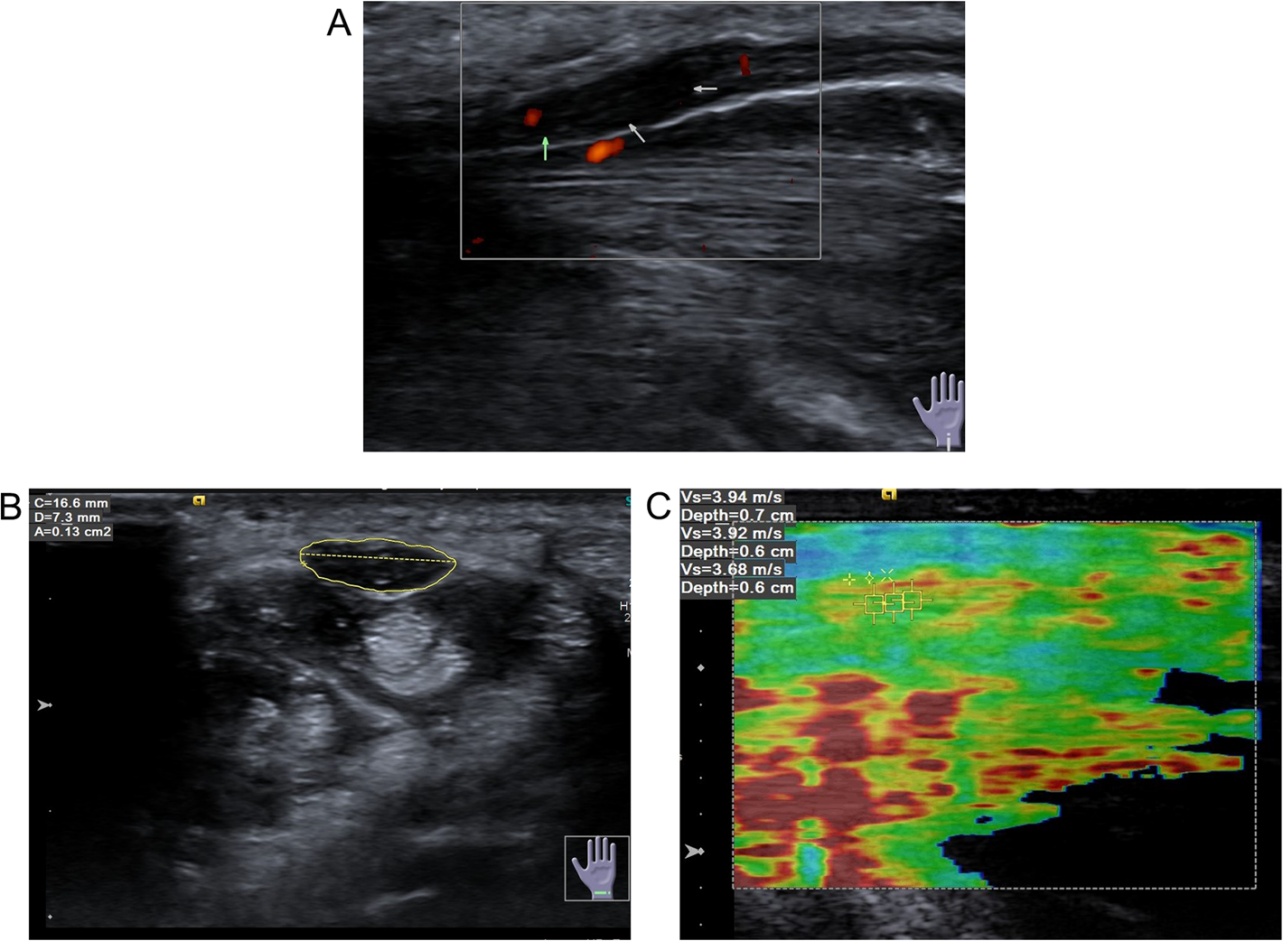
Ultrasound diagnosis (a) The longitudinal section of HFUS showed thickening of the middle normal nerve in carpal canal, decreased echo, and increased blood flow signal in power Doppler ultrasound; b Median nerve CSA was measured using the pisiform bone as a reference; c ROI was measured three times at the carpal tunnel inlet and outlet parallel to the pisiform bone and groove bone and different areas in the median nerve at the midpoint of the carpal canal
